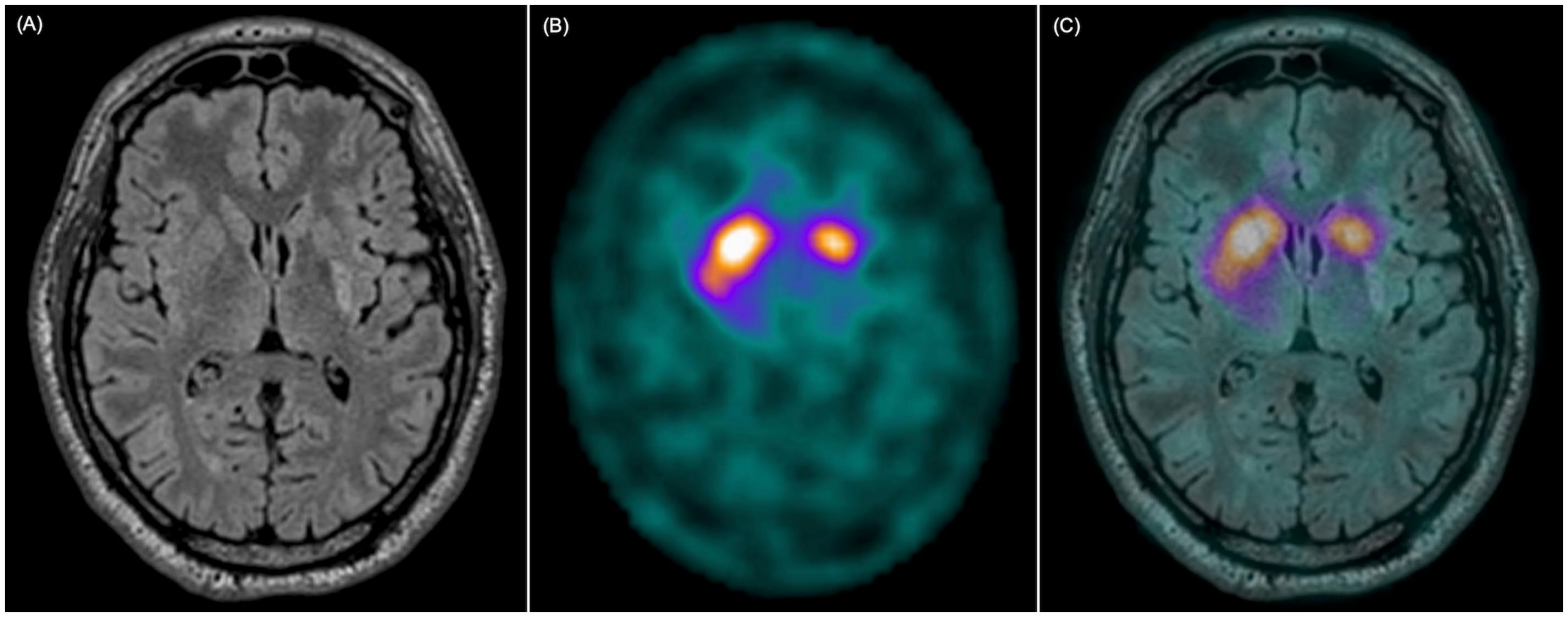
MRI and [^123^I]FP-CIT SPECT in a patient with an isolated striatal infarct. (A) T2-FLAIR weighted image shows infarct in the left posterior putamen. (B) [^123^I]FP-CIT SPECT shows reduced ligand uptake in the left posterior putamen. (C) Fusion of MRI and [^123^I]FP-CIT SPECT shows that the reduced ligand uptake closely follows the limits of the vascular lesion.
